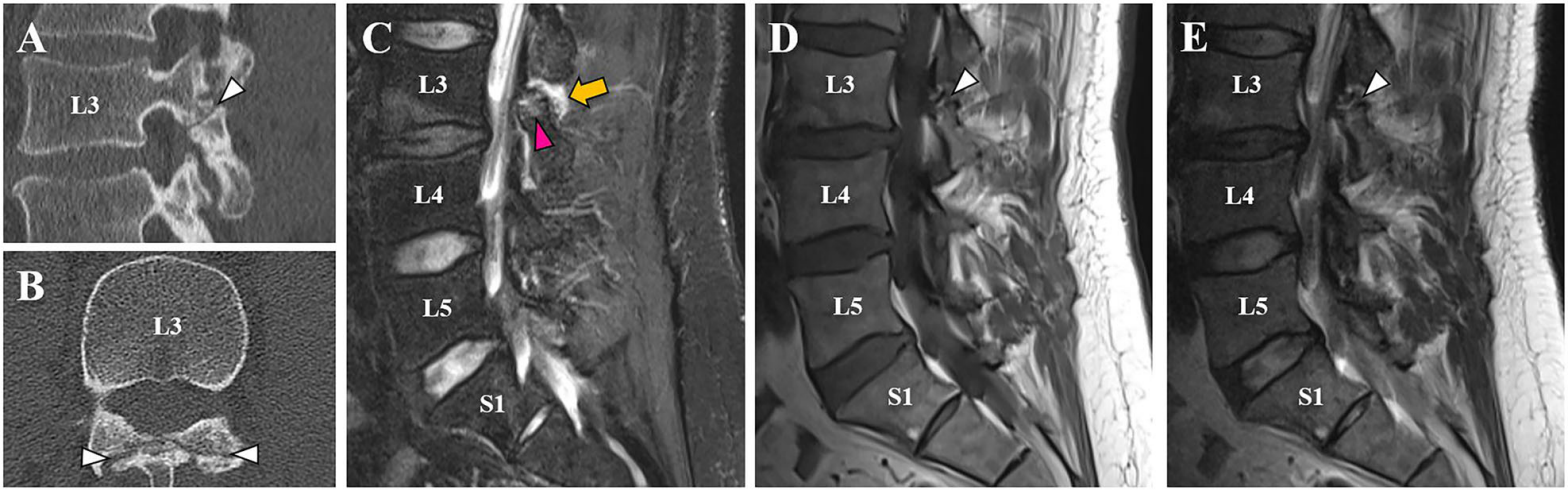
Forty-one-year-old male spondylolysis patient with CLBP, with 33% pain reduction at 15 min, and 100% pain reduction at 1-month follow-up after CT-guided bilateral pars injection. The sagittal (A) and axial (B) CT images show chronic bilateral spondylolysis at the L3 level with typical sclerotic margins of the lysis borders, which are also identified on the T2w TSE and T1w TSE images as hypointense bands along the pars defect (white arrowheads, A–E). Sagittal STIR images (E) reveal minimal isthmic BME (pink arrowhead) and adjacent soft tissue edema around the spondylolysis (yellow arrow). CLBP, chronic lower back pain; TSE, turbo spin echo
